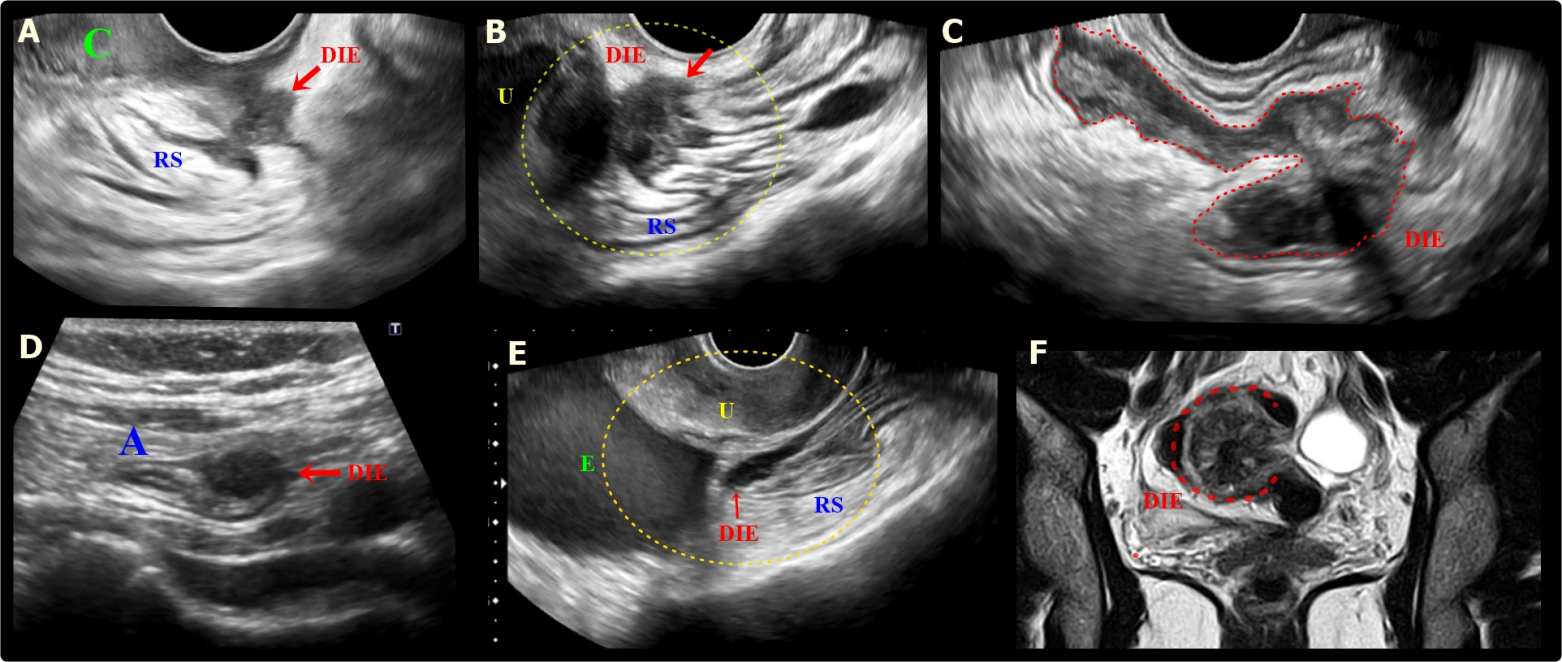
A. Sagittal transvaginal ultrasound (TVUS) image shows a hypoechoic endometriotic nodular lesion, with irregular margins, infiltrating the low retrocervical space, with extension to the posterior vaginal fornix and the serosa of the anterior rectal wall (RS) (red arrow). DIE: deep endometriosis. B. Sagittal oblique TVUS image shows a hypoechoic lesion (red arrow) with ill-defined margins, covering and infiltrating the posterior uterine wall and the anterior rectosigmoid wall (RS), causing retraction and angulation of this segment. U: Uterus. C. Sagittal oblique TVUS image shows a large hypoechoic endometriotic lesion in plaque (red dashed) infiltrating the anterior wall of the rectosigmoid colon. D. TVUS image demonstrates a hypoechoic nodule (red arrow) infiltrating the appendiceal tip (A). E. Sagittal oblique image of TVUS shows a hypoechoic endometriotic lesion infiltrating the retro- and paracervical space, with extension to the anterior wall of the rectosigmoid colon (RS) (red arrow). Also note, the presence of an ovarian endometrioma (E). U: Uterus. F. Coronal T2W MR image shows deep endometriosis lesion with archiform morphology in the rectosigmoid (red dashed).
